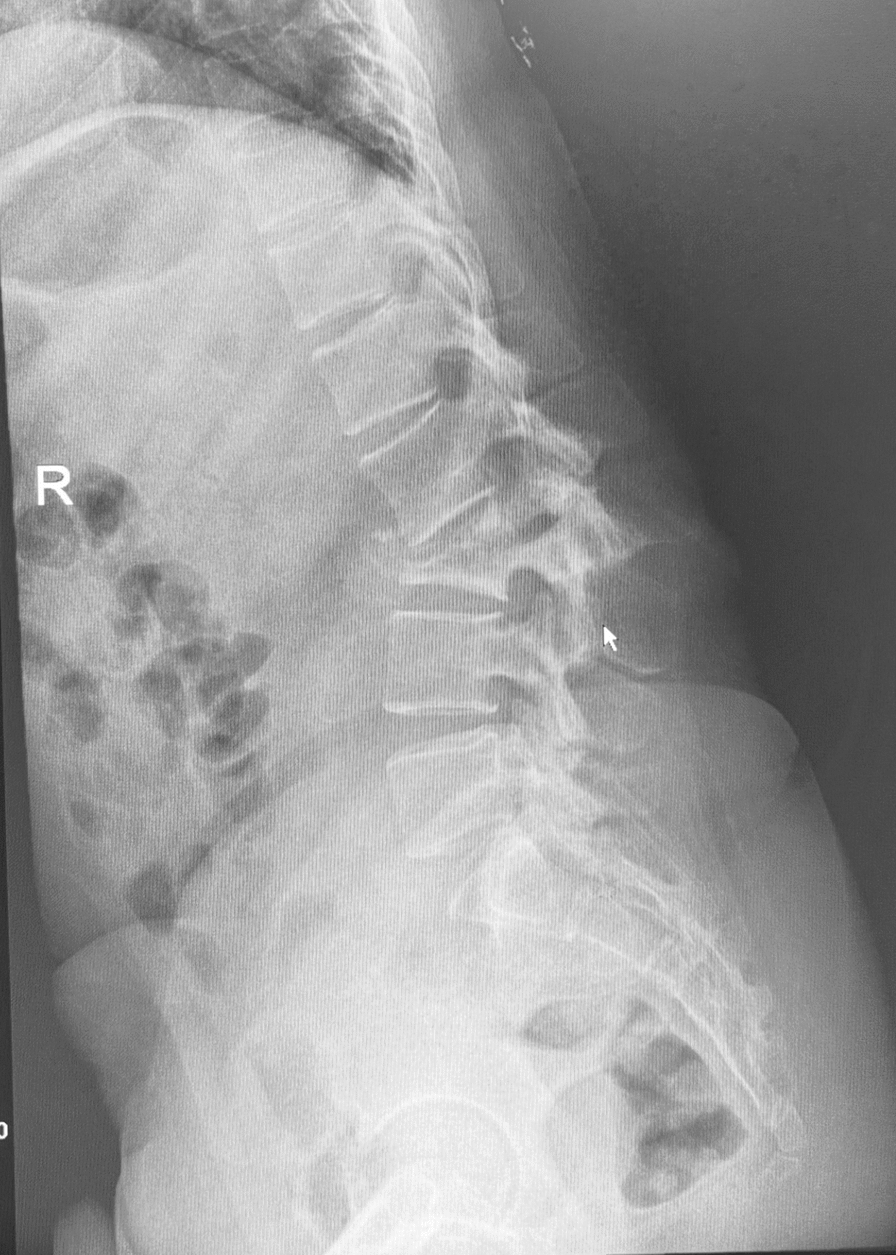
Lateral lumbar spine radiograph demonstrating a wedge-shaped compression fracture of L3 with focal kyphotic deformity and endplate irregularity. The loss of anterior vertebral height and endplate erosion are features compatible with tuberculous spondylodiscitis (Pott’s disease) in the appropriate clinical context. The white arrow indicates the collapsed L3 vertebral body and adjacent endplate changes
